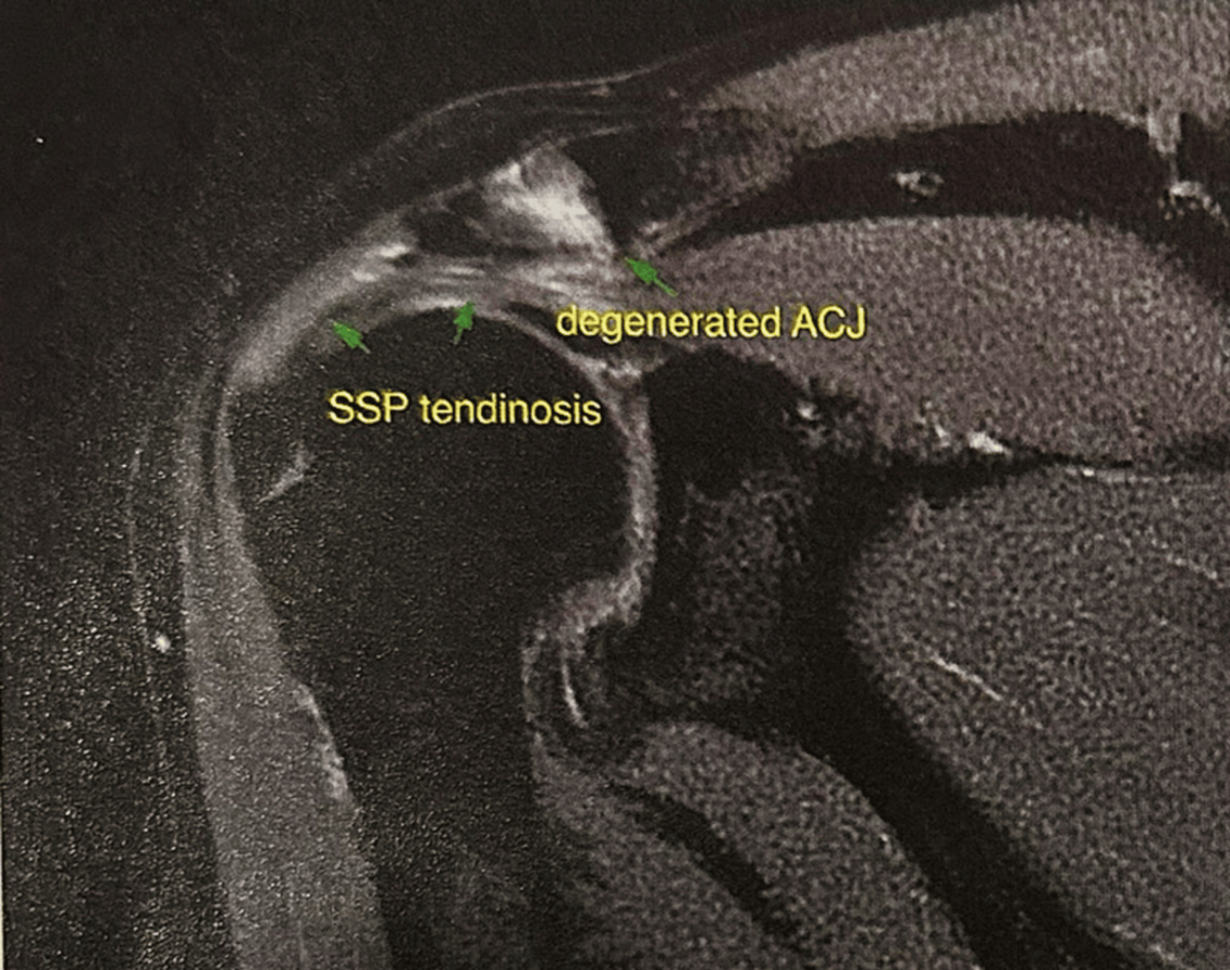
Coronal T2 fat sat magnetic resonance imaging (MRI) Showing a fluid-filled subacromial-subdeltoid (SASD) bursa with partial tearing of the supraspinatus (SSP) tendon at its insertion (green arrows). ACJ: acromioclavicular joint
